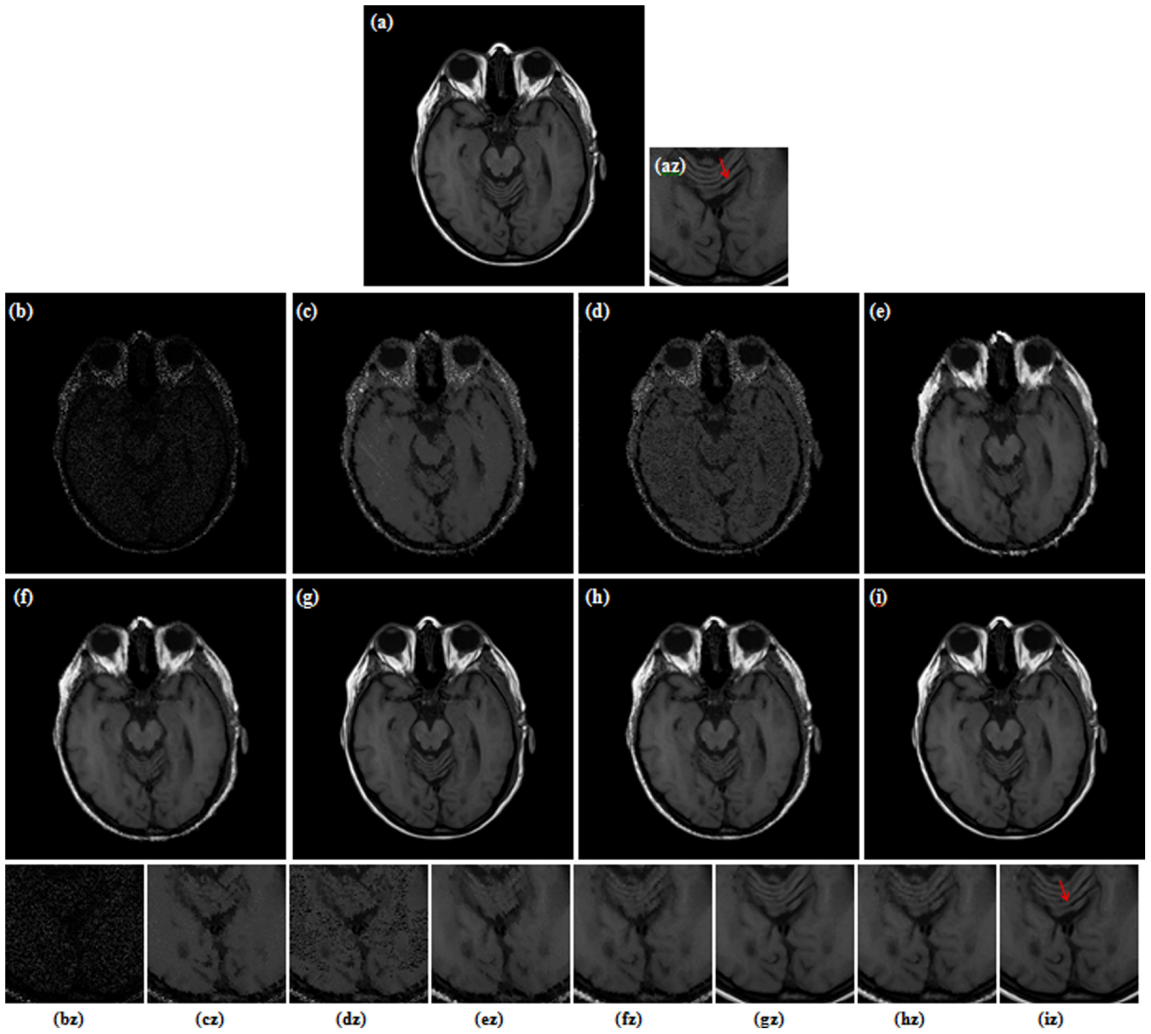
Restoration results for MRI image (80% salt-and-pepper noise). (a) Original image (b) Corrupted image (2.54 dB, SSIM = 0.0195). (c) AMF (8.53 dB, SSIM = 0.6889) [6]. (d) DBA filter (6.26 dB, SSIM = 0.6191) [7]. (e) MDBUTMF filter (13.68 dB, SSIM = 0.7981) [8]. (f) MDBUTAF filter (16.54 dB, SSIM = 0.8524). (g) SKR method (24.22 dB, SSIM = 0.9203) [23]. (h) RGMLE-C algorithm (19.74 dB, SSIM = 0.9012). (i) RGMLE-CS algorithm (23.76 dB, SSIM = 0.9226). (az)–(iz) denote the zoomed local parts for the corresponding images in (a)–(i).
